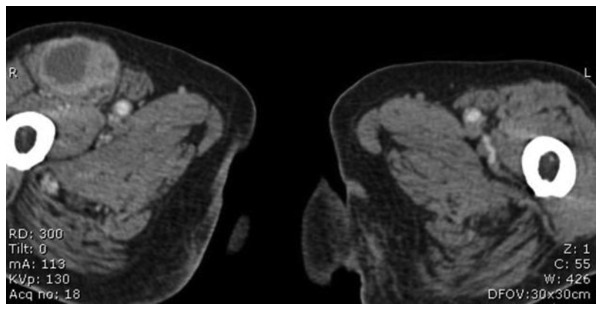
CT scan showing a solid expansive lesion in the rectus femoris muscle.
